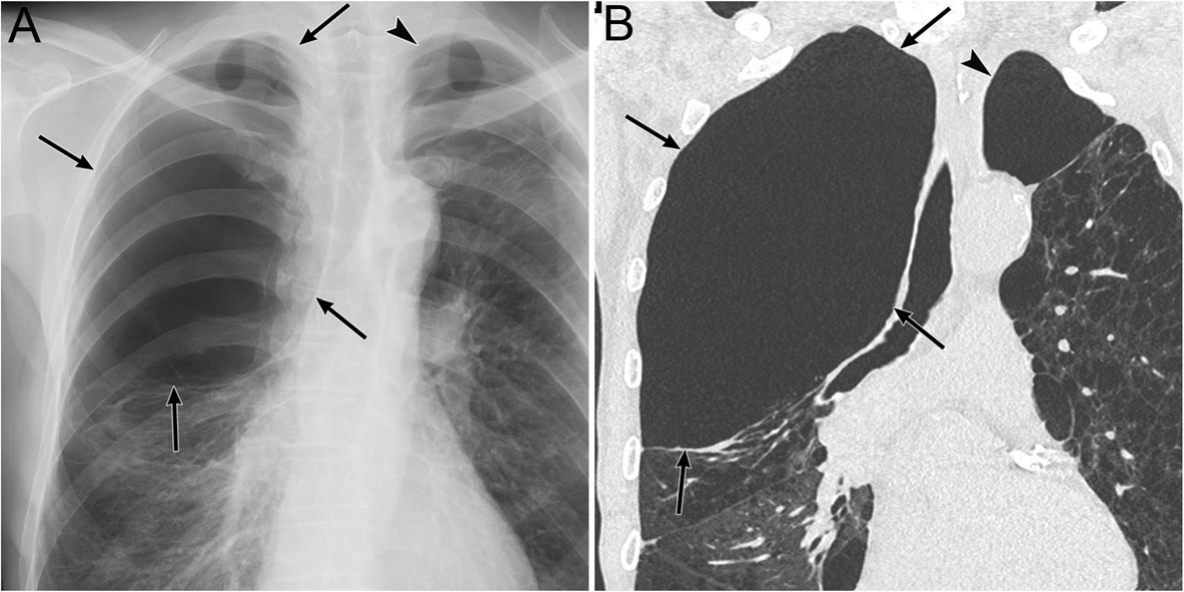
A giant bulla mimicking pneumothorax on a radiograph in a 67-year-old man. He has chronic obstructive pulmonary disease and a cigarette smoking habit. a A giant bulla appears as an oval radiolucency (black arrows) in the right lung apex on an erect radiograph. It resembles pneumothorax in the apicolateral space. Bullous emphysema exists in both lungs, and a large bulla is observed in the left lung apex (arrowhead). b A coronal CT image reveals a giant bulla (black arrows) in the right lung apex. A large bulla (black arrowhead) also exists in the left lung apex in addition to bullous changes in both lungs
